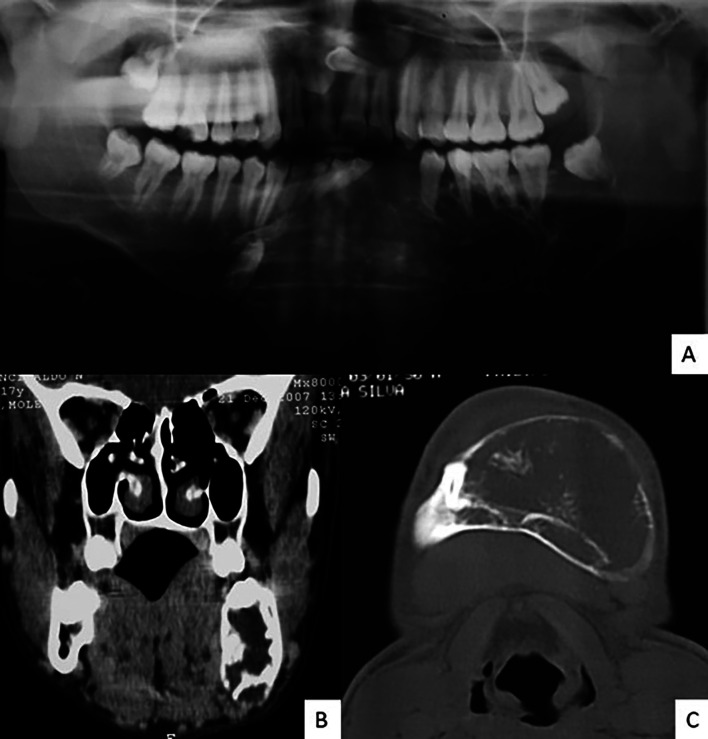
A) Panoramic radiograph showing a multilocular image, poorly defined, extensive in the bilateral anterior and posterior regions of the mandible on the left, radiolucent with radiopaque intralesional trabeculae, displacement of mandibular anterior teeth on the right side, tooth 33 retained in the right mandibular base, root resorption of teeth 34, 36 and 37. Computed tomography in B) coronal section showing hypodense image causing bone expansion in the posterior mandibular region on the left with bone fenestration in the lingual and basal cortical; and C) axial section showing multilocular hypodense image causing mandibular expansion, with hyperdense areas inside, with preservation of the lingual cortex.
